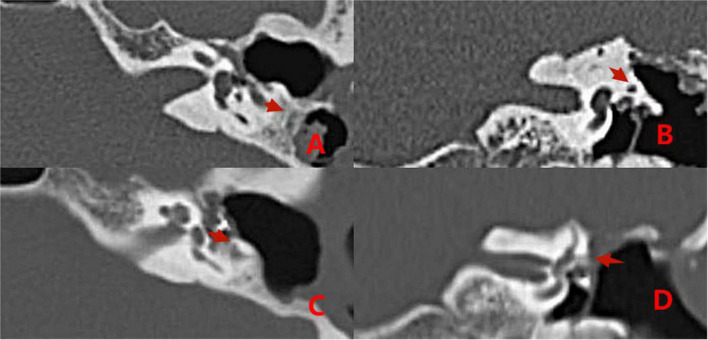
CT images acquired before and after surgery from a patient with type II labyrinthine fistula undergoing a second surgery. Preoperative (A,B): The left cholesteatoma is adjacent to the bone resorption with a normal external semicircular canal shape (the red arrows). Postoperative (C,D): The removal of cholesteatoma, the abnormal shape of the external semicircular canal, and the intraoperative fillers inside and adjacent to it (the red arrows).
